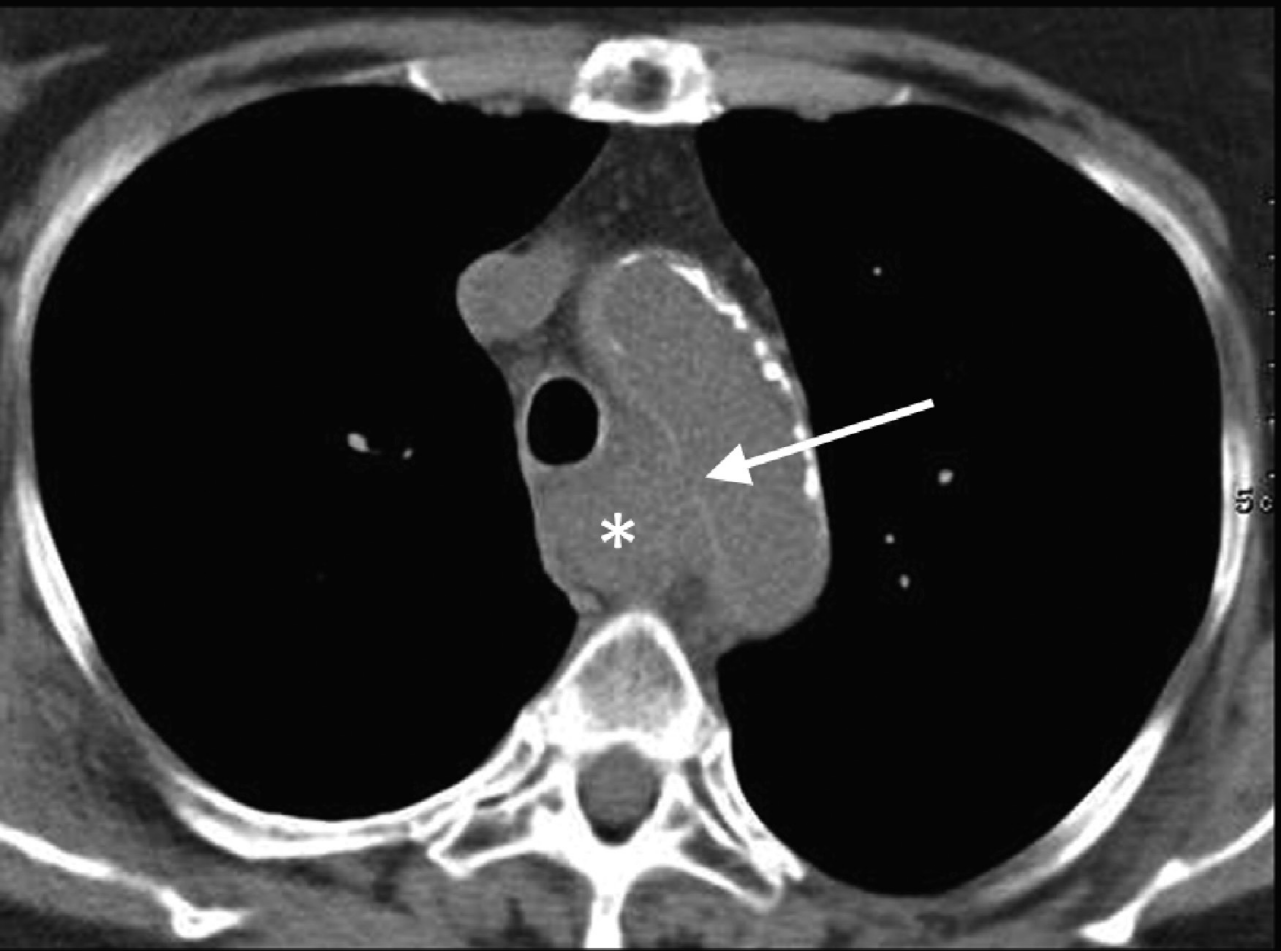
Unenhanced CT showing a retrotracheal, paraortic hyperdense mass (star). There is a small discontinuity in the hyperdense aortic wall, representing the point of fistulation (arrow).
